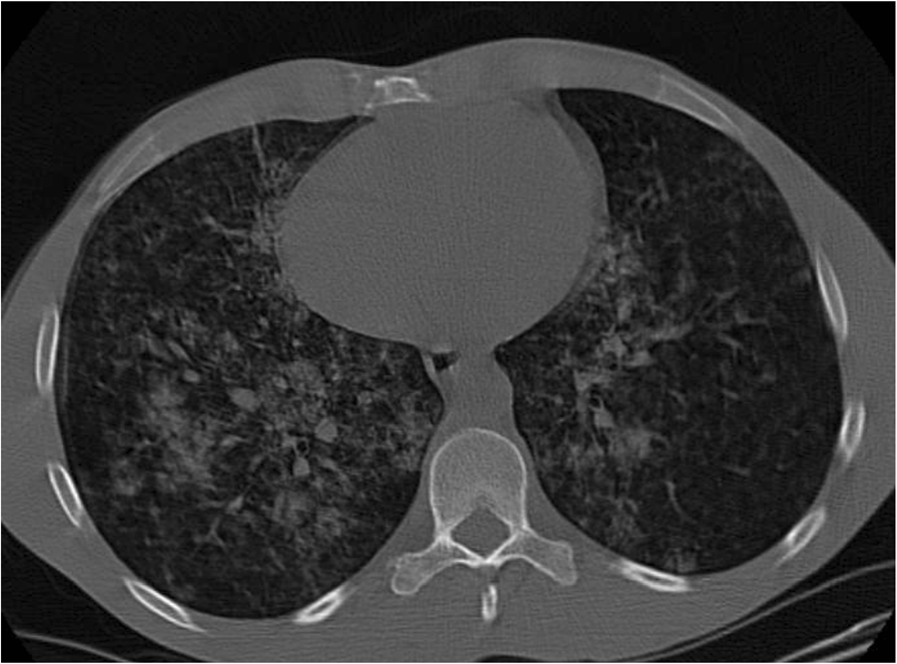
Chest computed tomography scan. The chest computed tomography scan showed bilateral multilobular diffuse infiltrates
